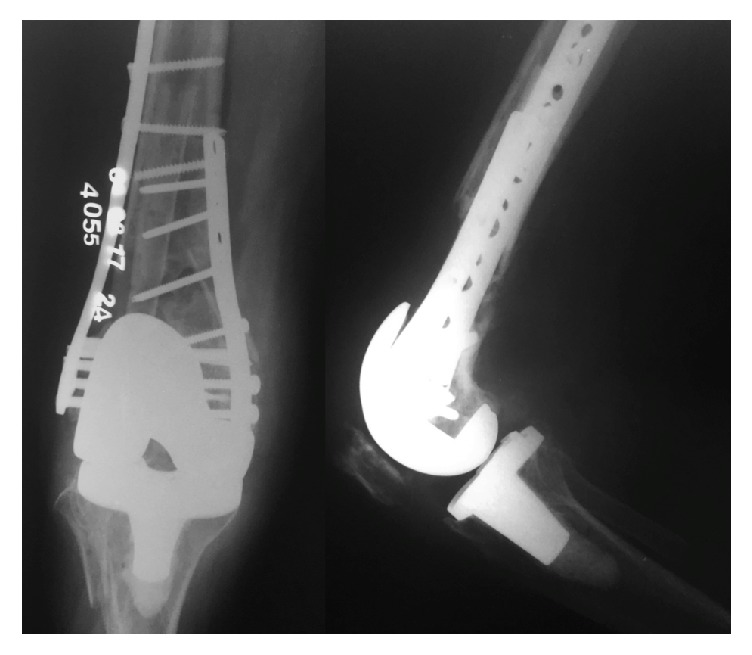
Final follow-up radiographs showing osseointegration of the fibular graft with definite bridging of the distal metaphyseal femur defect.
